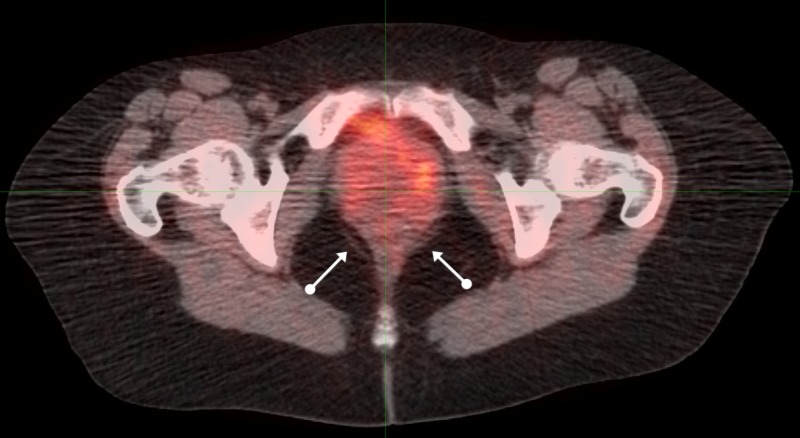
Sequential axial PET/CT images of pelvic malignancy: distal positron emission tomography-computed tomography: PET/CT
